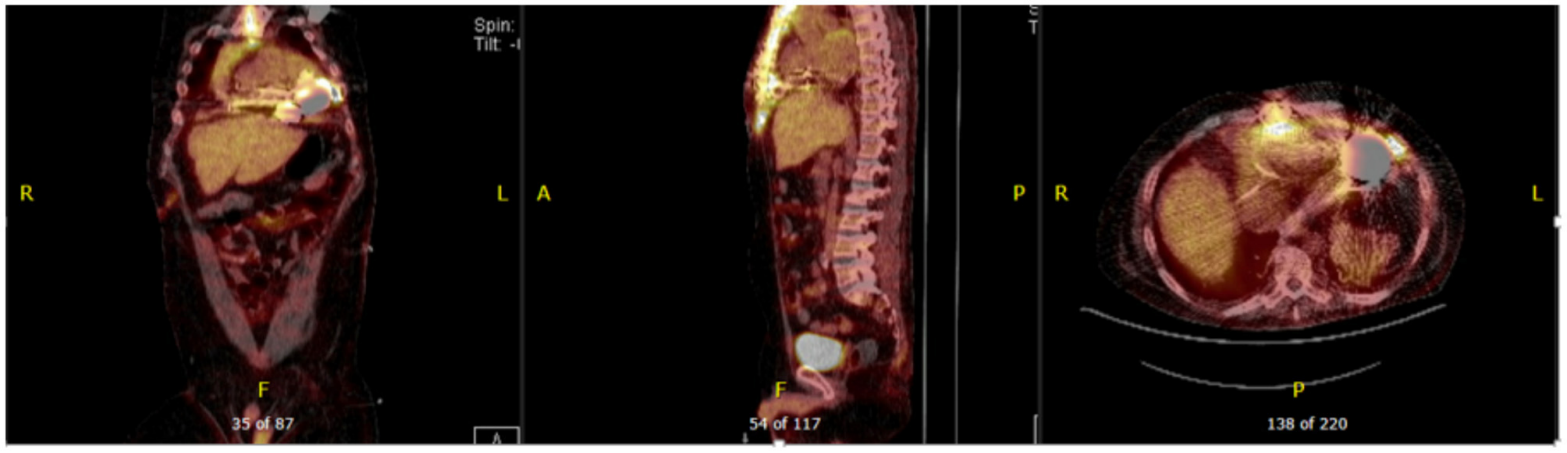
Forty seven year-old male with ischemic cardiomyopathy s/p LVAD (Heart Mate 3) presented with suspected DLI, with wound cultures positive for Candida Albicans. ^18^F-FDG PET-CT showed FDG activity in the pericardium, mediastinum, surrounding LVAD pump, driveline and sternum consistent with deep and superficial LVAD infection. He was managed with systemic lifelong antifungal therapy.
